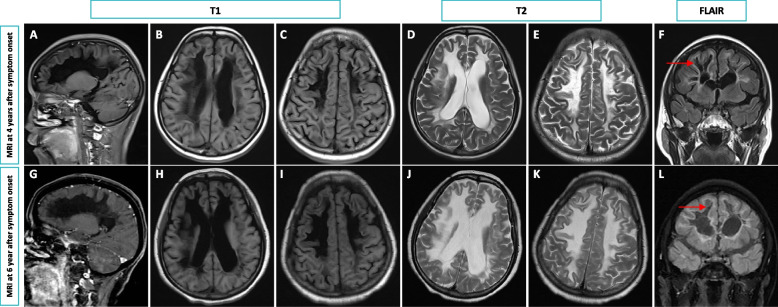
MRI changes of the patient at two time points. MRI obtained at 4 years (A-F) and 6 years (G-L) after the onset of the symptoms. Hypointense signal was observed on sagittal T1-weighted MRI (A, G). Axial MRI showed extensive, symmetric, confluent hyperintensities in the white matter, predominantly in the periventricular (B, D, H, J) and frontoparietal (C, E, I, K) regions, on the T1-weighted MRI (B, C, H, I) and hypointensities on the T2-weighted MRI (D, E, J, K). Coronal FLAIR images (F and L) revealed the affected white matter adjacent to the anterior and posterior horns of lateral ventricles had a signal intensity identical to that of CSF, which was suggestive of cystic degeneration (Red arrow)
